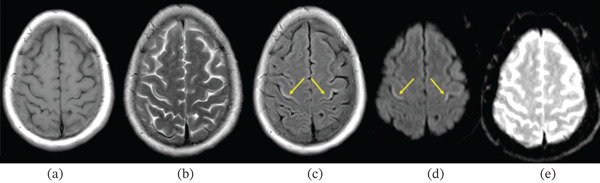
The bilateral precentral hyperintensity is better appreciated on (c, d) FLAIR and DWI images, marked by yellow arrows, and is seen as (a, b) faint T1 hypointensity and T2 hyperintensity, with no associated drop on (e) ADC map. This figure highlights the importance of FLAIR images in identifying this subtle lesion.
